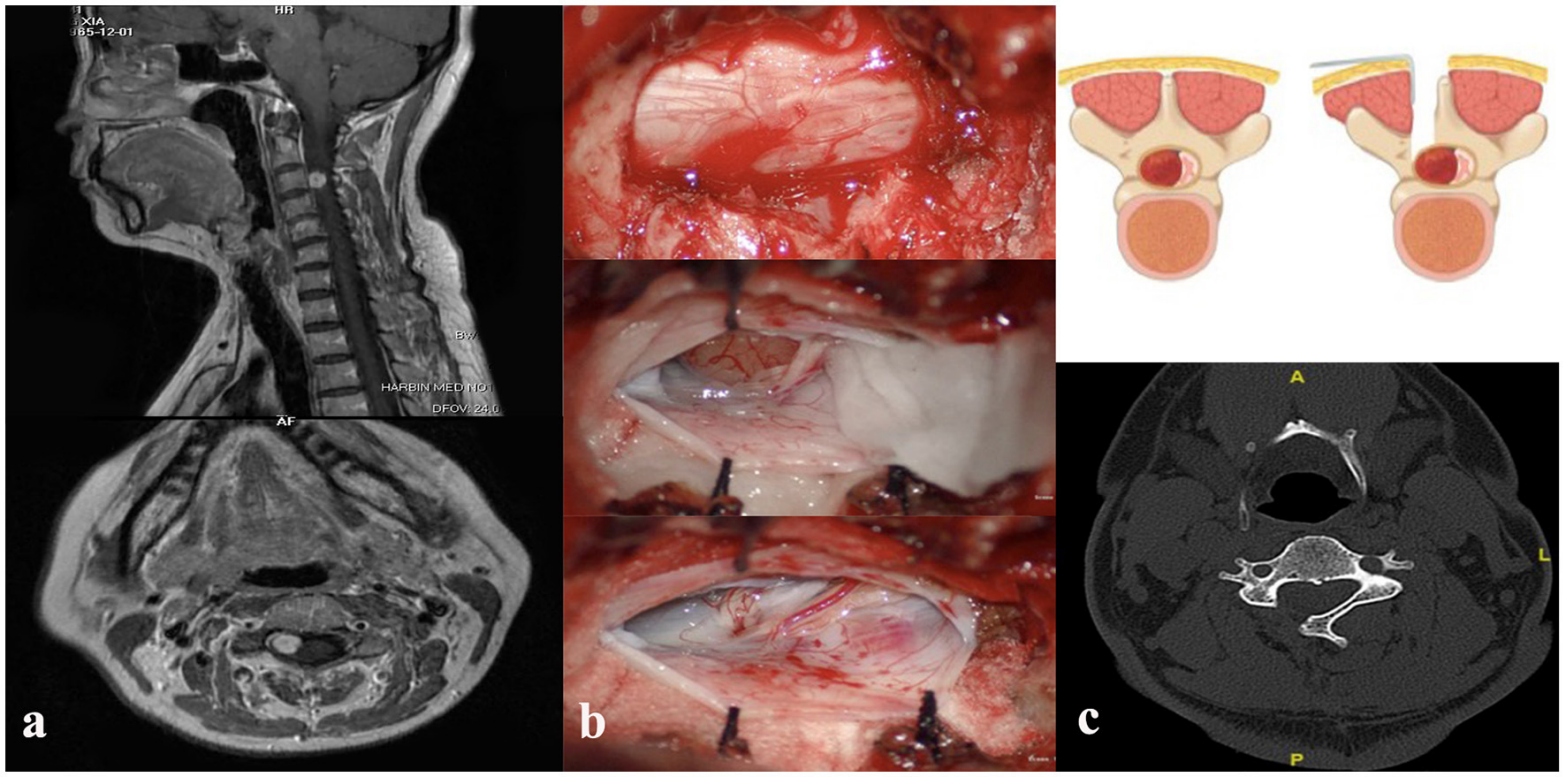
(a) Preoperative cervical magnetic resonance imaging T1-weighted sagittal (up) and axial (down) image with contrast showing heterogeneously enhancing intradural extramedullary lesion at the level of the C3 vertebrae. (b) Intraoperative unilateral hemilaminectomy with resection of the laminae (up), exposure of the tumor (middle), and removal of the lesion (down). (c) Demonstration of unilateral hemilaminectomy (up), postoperative computed tomography scan study (down).
